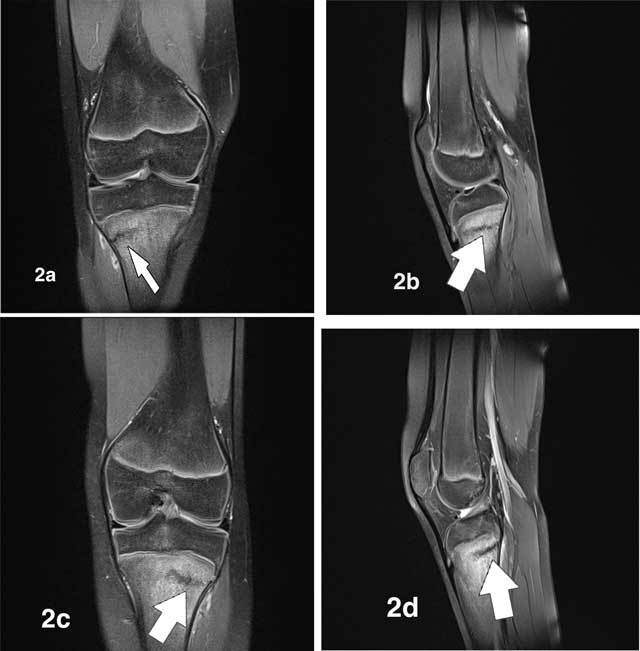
On fatsuppressed (FS) T2-weighted fat-saturated MR images, the fracture lines (white arrows) are of low signal and are surrounded by an extensive high-signal area of bone marrow oedema. a. Coronal FS T2-weighted image (WI) of the right knee. b. Sagittal FS T2-WI of the right knee. c. Coronal FS T2-WI of the left knee. d. Sagittal FS T2-WI of the left knee.
